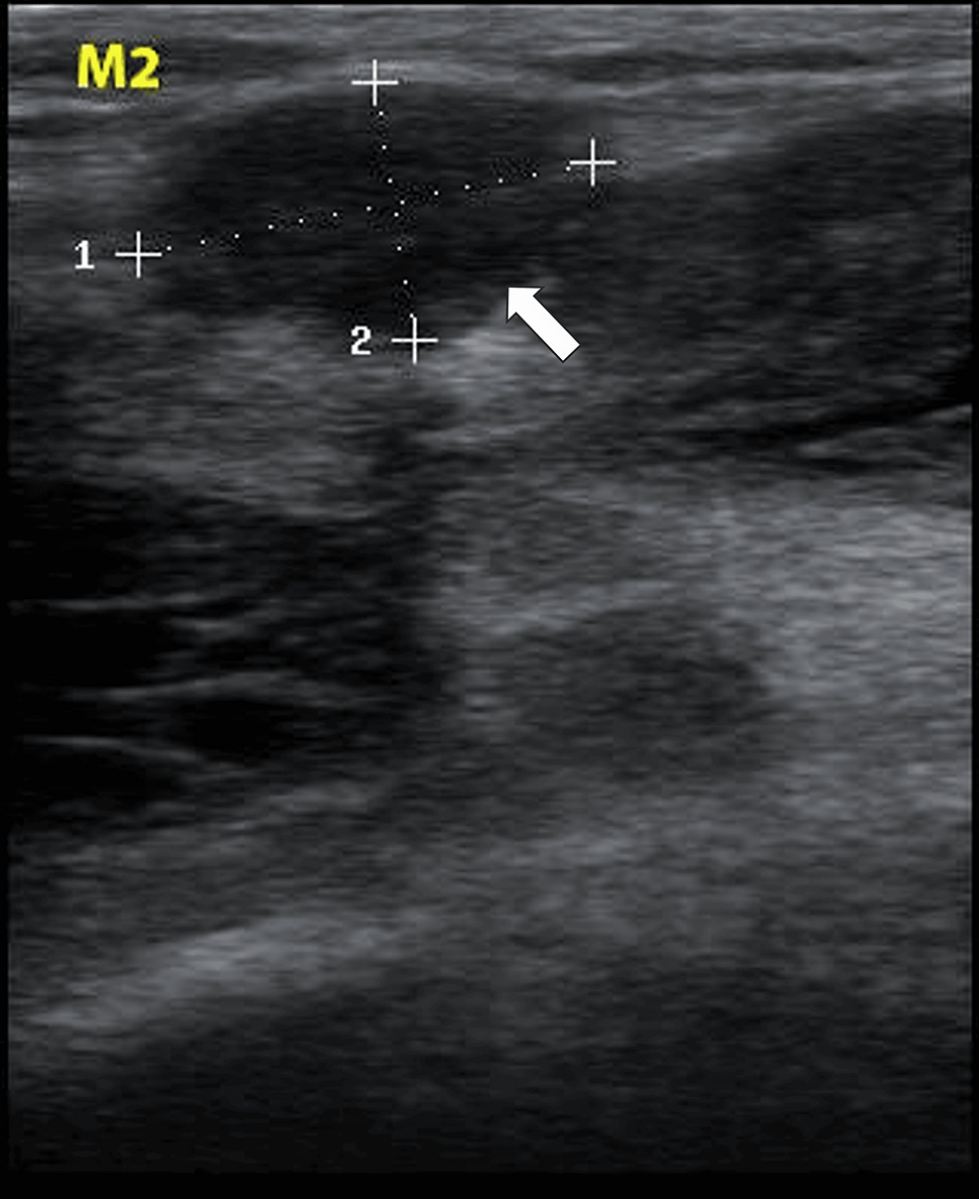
Ultrasound image of M2 The image shows a mass located in the suprapatellar compartment behind the tendon sheath of the vastus lateralis, measuring 1.81 cm (dotted line 1) x 1.02 cm (dotted line 2)
